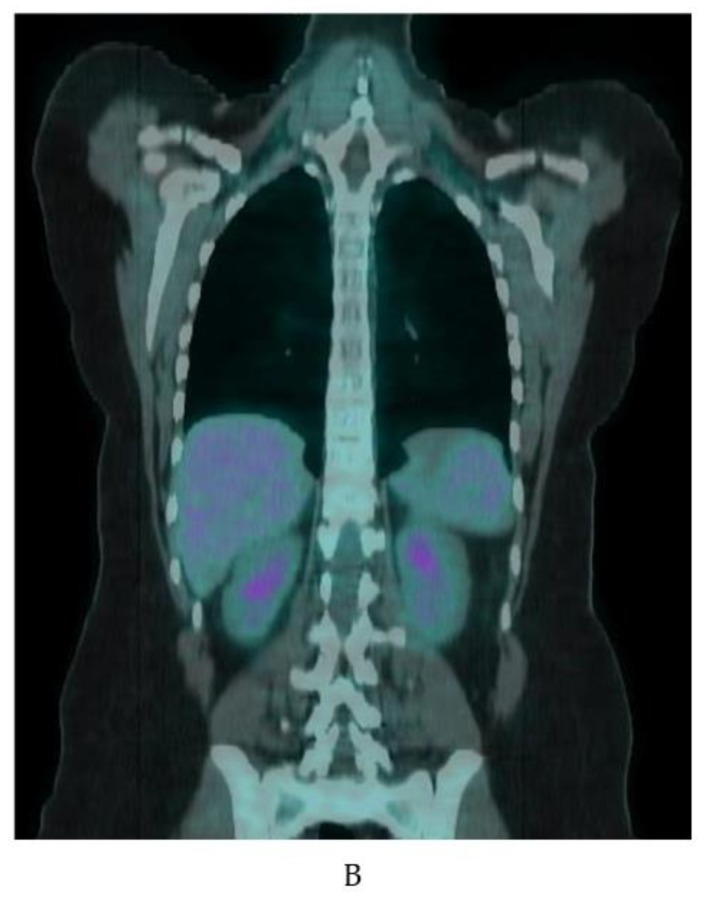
Example of variable iBAT uptake on FDG-PET/CT. FDG-PET/CT in a woman in her 20s showing marked BAT uptake in (A) November (yellow arrows) and no detectable BAT FDG uptake on a scan (B) 6 months later.
